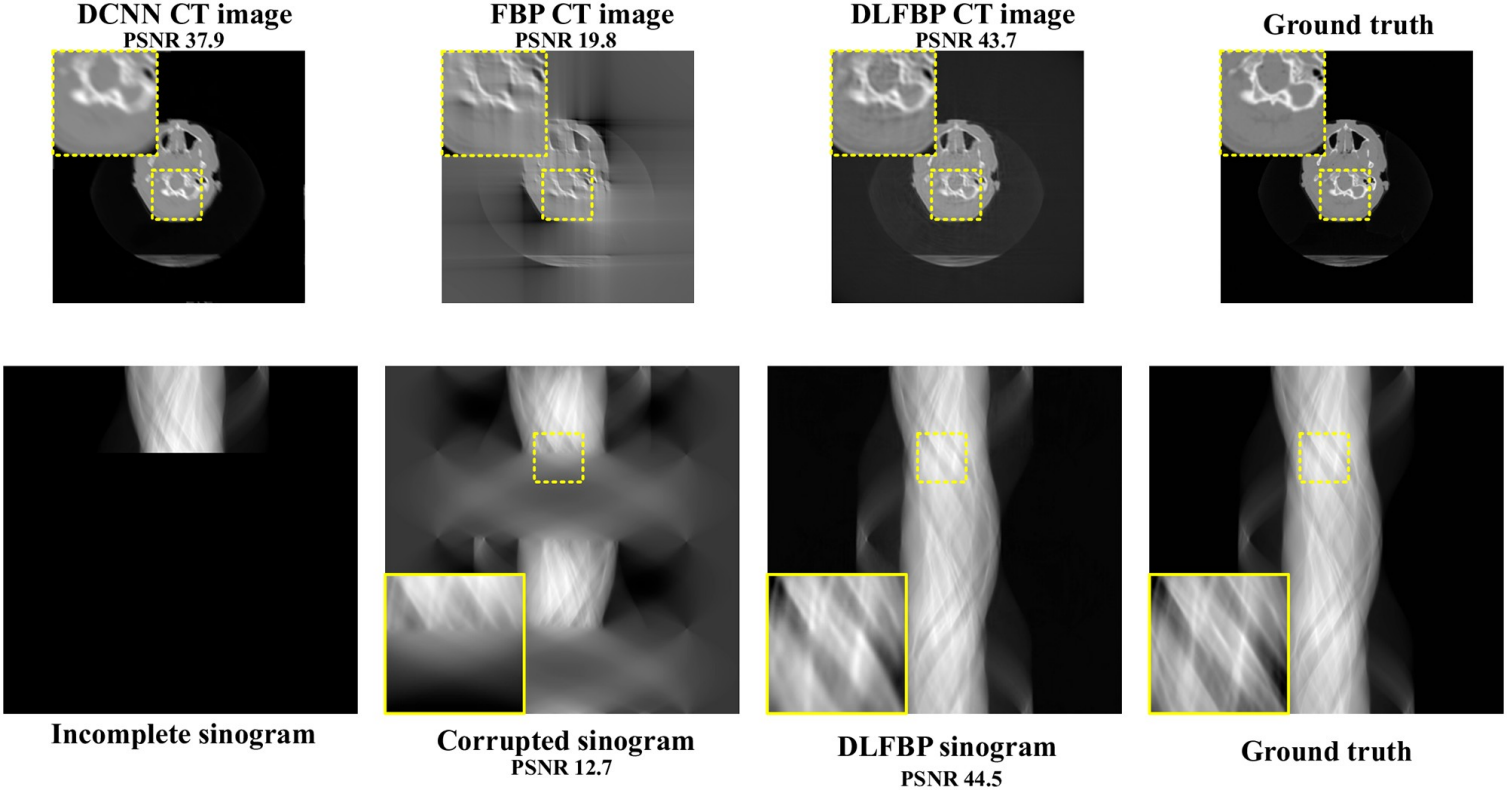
The limited-angle results of one head slice with the projections within the angular range [0 90°] by using DCNN [32], FBP and DLFBP. The incomplete singoram has a size of 180 × 731 pixels. The corrupted sinograms generated by the forward projection have a size of 720 × 731 pixels. All CT images have a size of 512 × 512 pixels after cutting off the surrounding blank region. Some regions of these images, indicated by the yellow box, are enlarged for better visualization.
